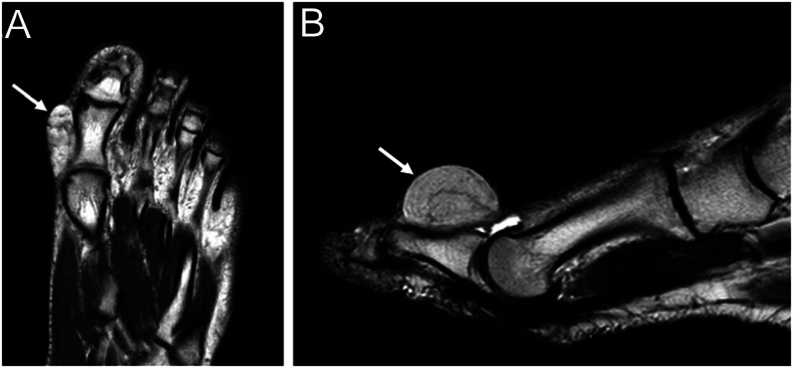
Imaging appearance of superficial left-foot lipoma of first ray (white arrows), hyperintense homogeneous signal on T1-weighted MRI in (A) transverse and (B) sagittal projection.
